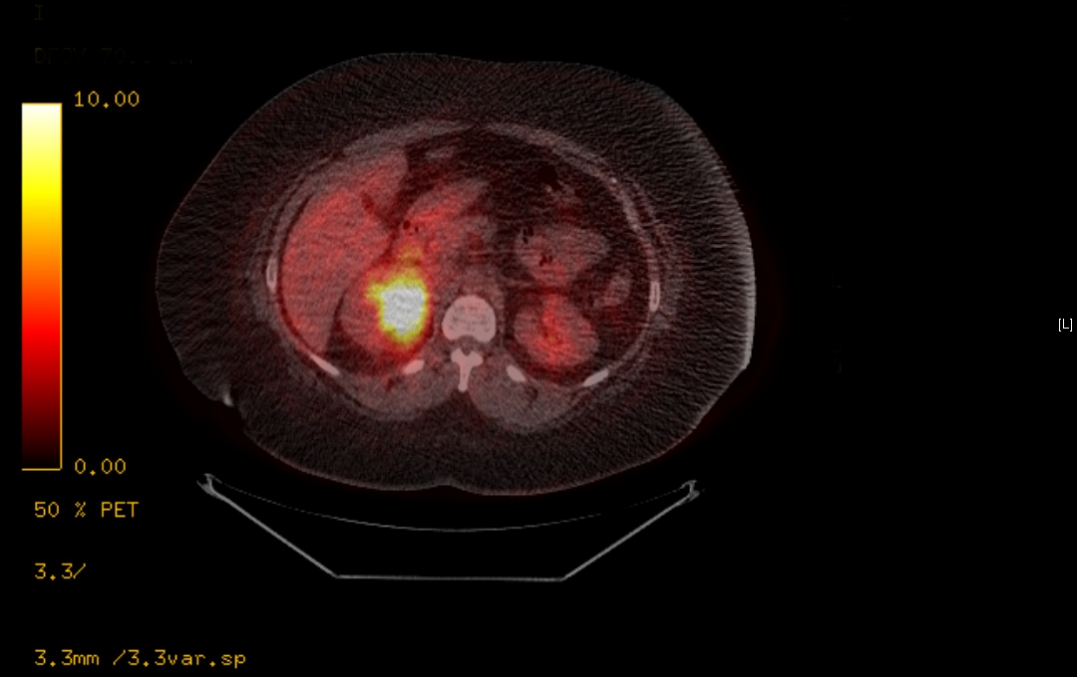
Preoperative PET-CT of the abdomen showing large right adrenal mass that is markedly hypermetabolic with peak SUV of 15.0.
